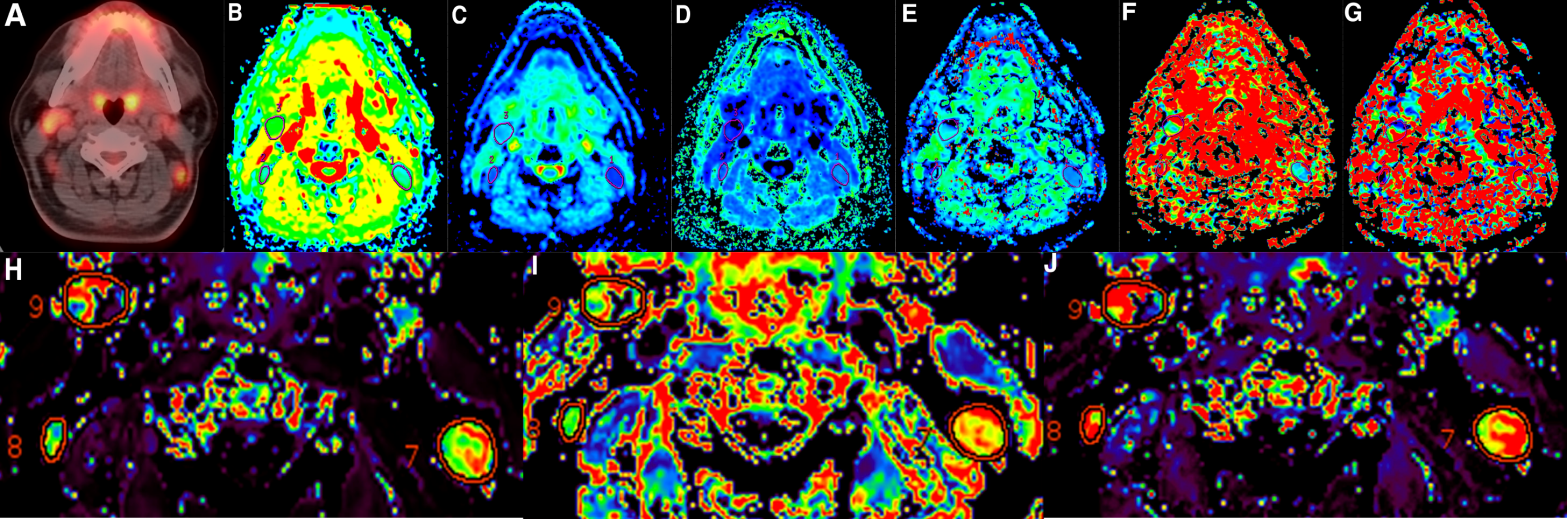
A 34-year-old man diagnosed with nasopharyngeal carcinoma has underwent PET-CT and multiparameter functional MR examinations. (A) PET/CT before induction chemotherapy (IC) confirmed the metastatic lymph nodes located in IIa and IIb, (B) apparent diffusion coefficient (ADC), (C) mean diffusion coefficient (MD), (D) mean kurtosis (MK), (E) pure molecular diffusion coefficient (D_slow_), (F) pseudo-diffusion coefficient (D_fast_), (G) perfusion fraction (PF), (H) volume transfer constant (K^trans^), (I) volume fraction of extravascular extracellular space (V_e_), and (J) the reflux rate constant between extravascular–extracellular space and plasma (K_ep_) maps derived from functional MRI (fMFRI) before induction chemotherapy (IC).
